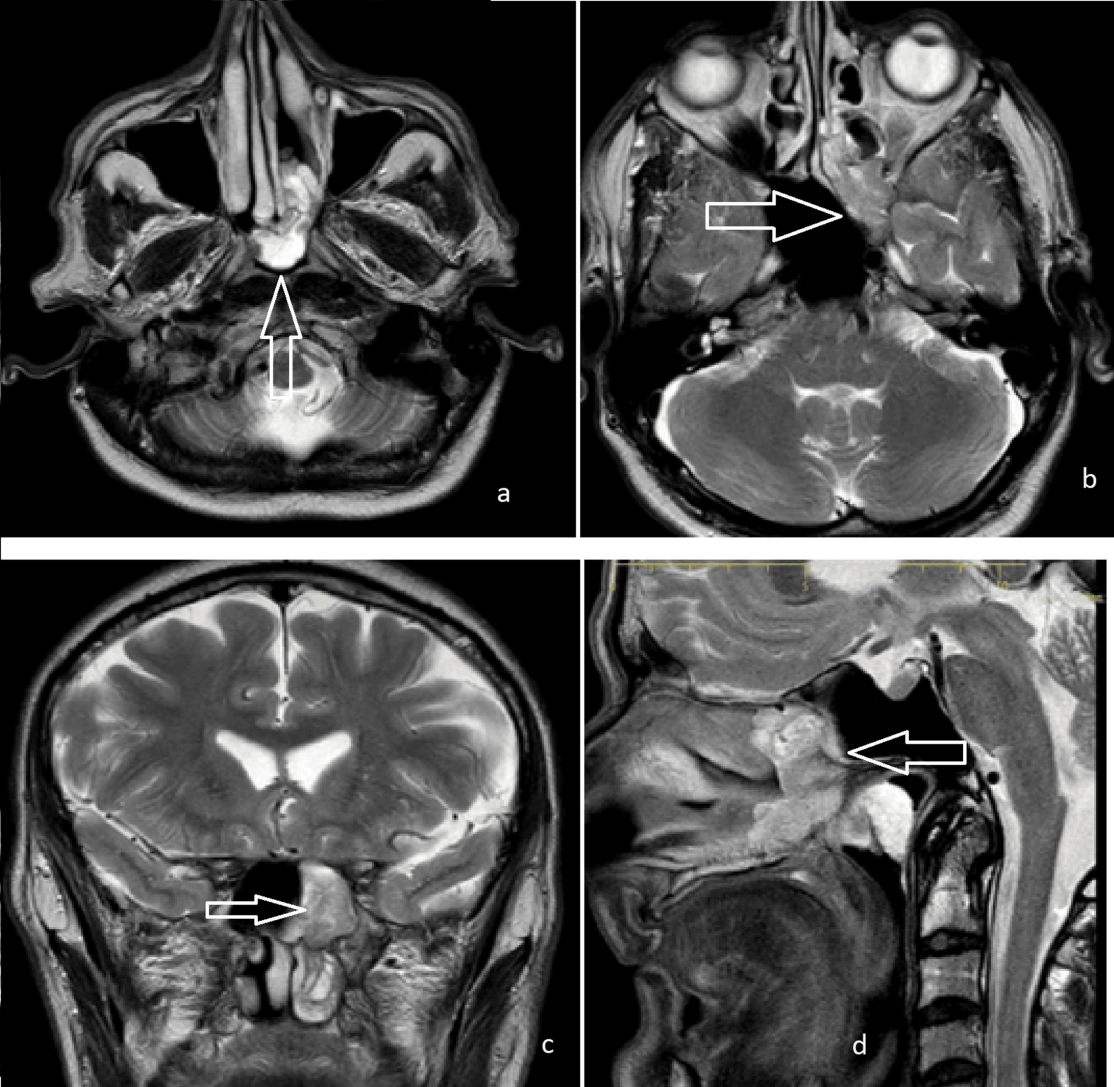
MRI scan images of the patient with isolated sphenoid (a,b) axial, (c) coronal, (d) sagittal views.
